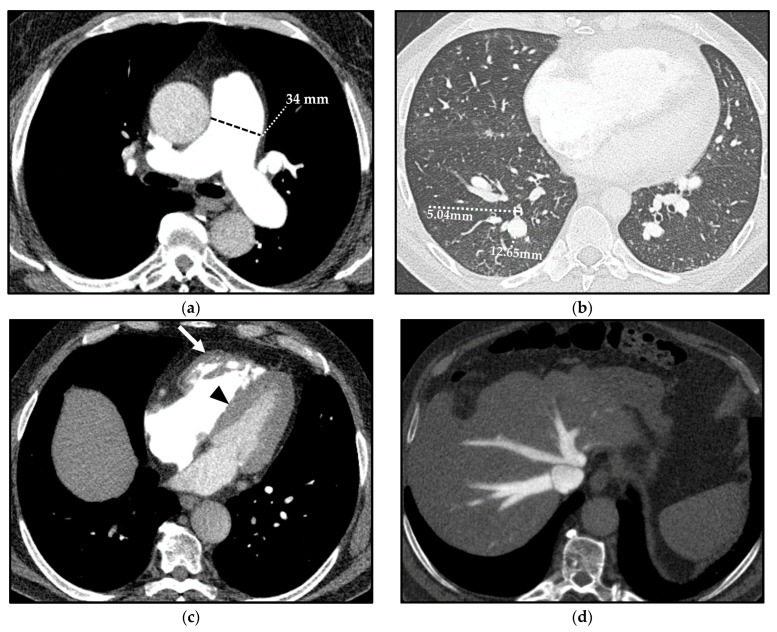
Computed tomography (CT) indirect signs of pulmonary hypertension (PH). Vascular CT signs: main pulmonary artery (MPA) dilation (34 mm) (a) and increased segmental artery-to-bronchus ratio (>1) (b). Cardiac CT signs: right ventricle (RV) hypertrophy, with free wall thickness > 4 mm and trabecular hypertrophy (arrow), and flattening of the ventricular septum (arrowhead) (c). Extensive reflux of contrast medium into the inferior vena cava and hepatic veins (d).
